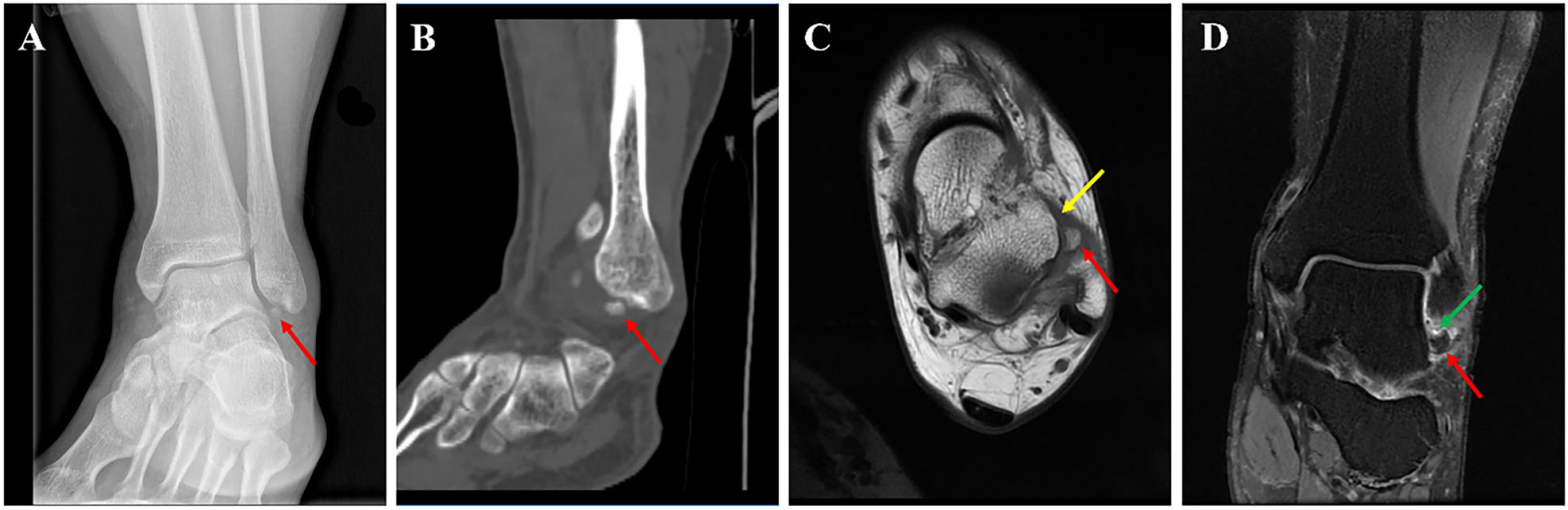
Imaging findings in a 29-year-old male OSF patient. A Ankle anteroposterior radiograph: the SFO (red arrow) demonstrates an incongruent relationship with the lateral malleolus. B Sagittal CT reconstruction (bone window): the SFO (red arrow) exhibits an irregular contour, cortical irregularity, and an anteroposterior long-axis orientation. C Axial T1WI: the SFO (red arrow) is located anteromedial to the lateral malleolus and contacts the ATFL (yellow arrow). D Coronal PDWI: intervening T2-hyperintense signal is present between the SFO (red arrow) and the fibula (green arrow), and the ossicle itself shows no evidence of associated marrow edema
